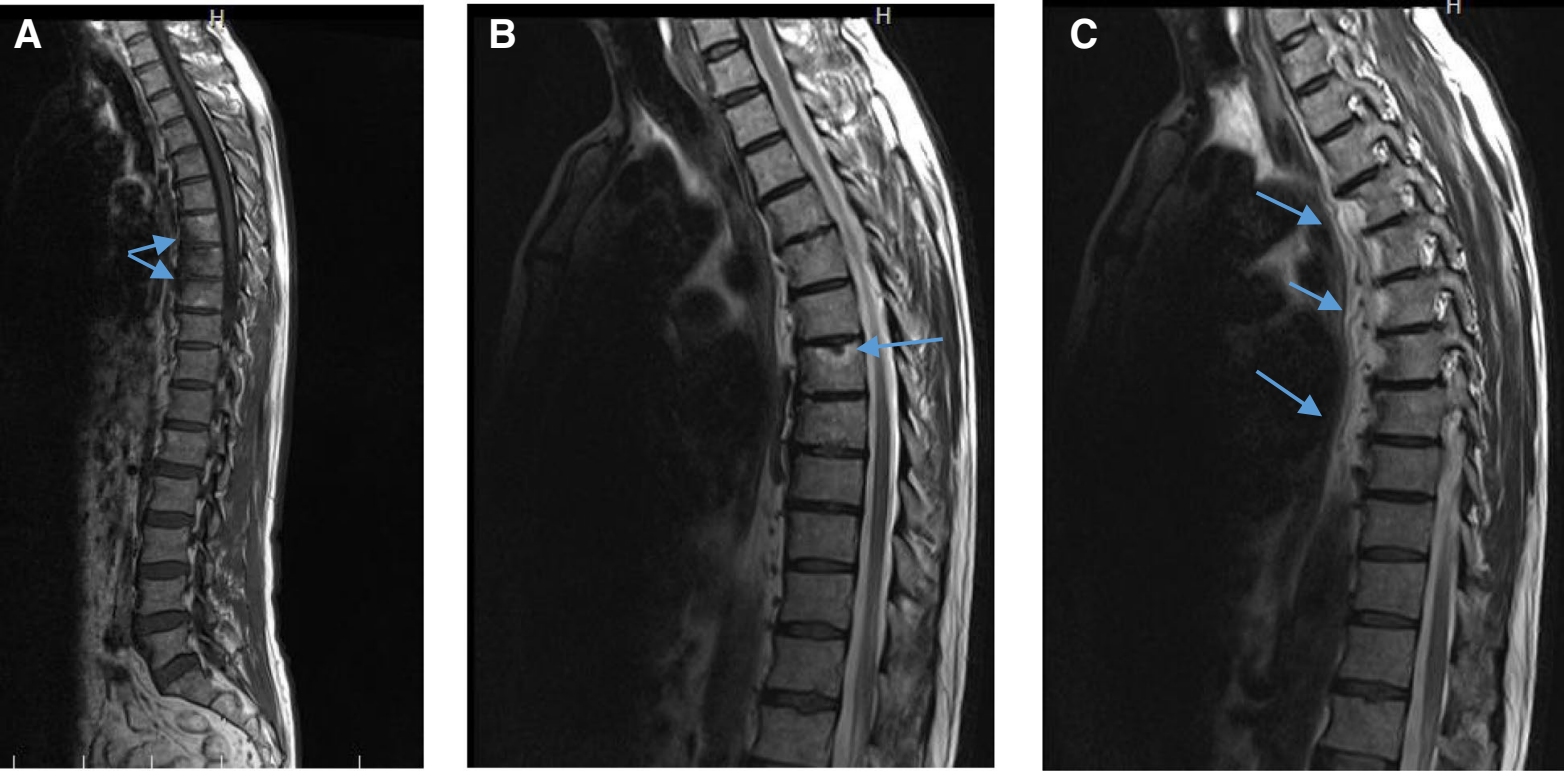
T1- and T2-weighted sagittal spinal MRI. T2-weighted sagittal MRI reveals bone marrow edema (osteitis) of 7th and 8th thoracic vertebrae in parallel with bone scan findings (A). T1-weighted MRI showing cortical erosion with surrounding edema in 7th thoracic spinal vertebra (B). Flowing thick osteophytes along anterior border of thoracic vertebrae without intervertebral disk involvement consistent with DISH (C). MRI, magnetic resonance imaging; DISH, diffuse idiopathic skeletal hyperostosis. All radiographic images were screenshotted from the compact discs.
